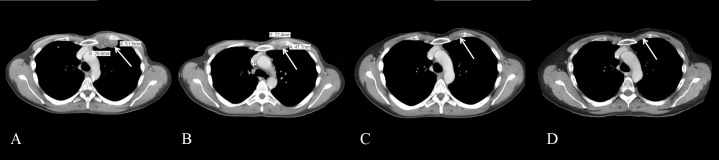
CT Chest with Intravenous Contrast Over Time Images taken three weeks before (A), two months into (B), five months into (C), and nearly six years (D) into treatment for metastatic disease, showing resolution of left chest wall mass
